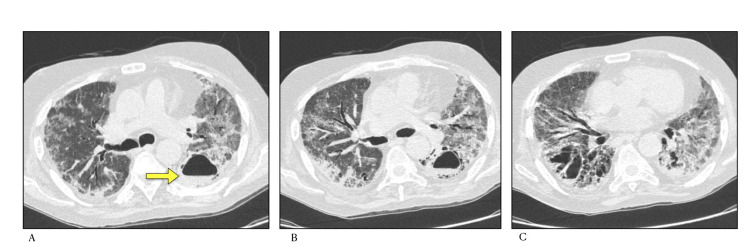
HRCT Chest (lung window) axial scans A), B) and C) demonstrate a cavity with an air fluid level in left lower lobe (arrow) with background changes of organizing consolidation and fibrosis
